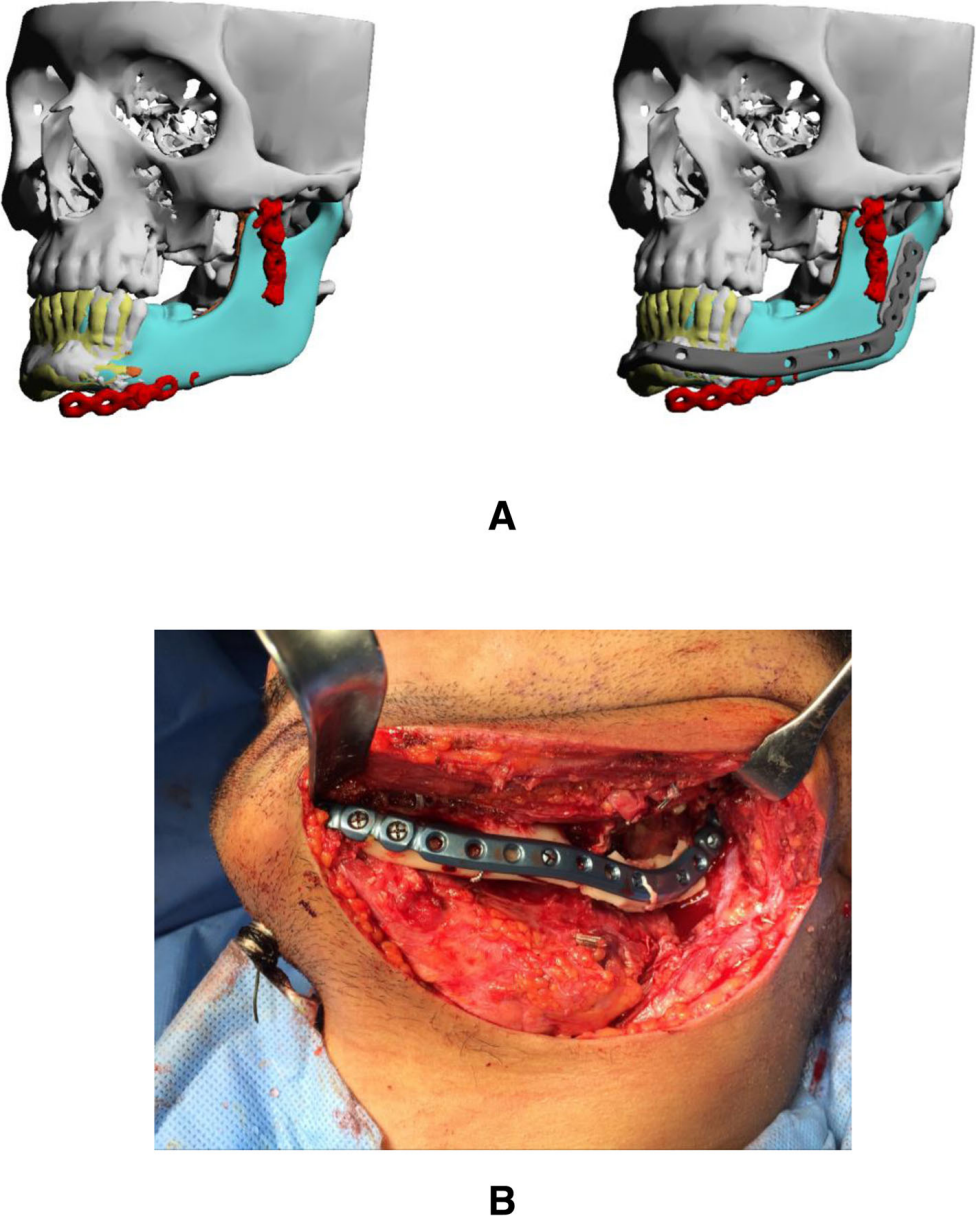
Post-free flap reconstruction case. a 3D reconstruction of CT showing previous plate and planned custom titanium implant. b Intraoperative view of the implant
